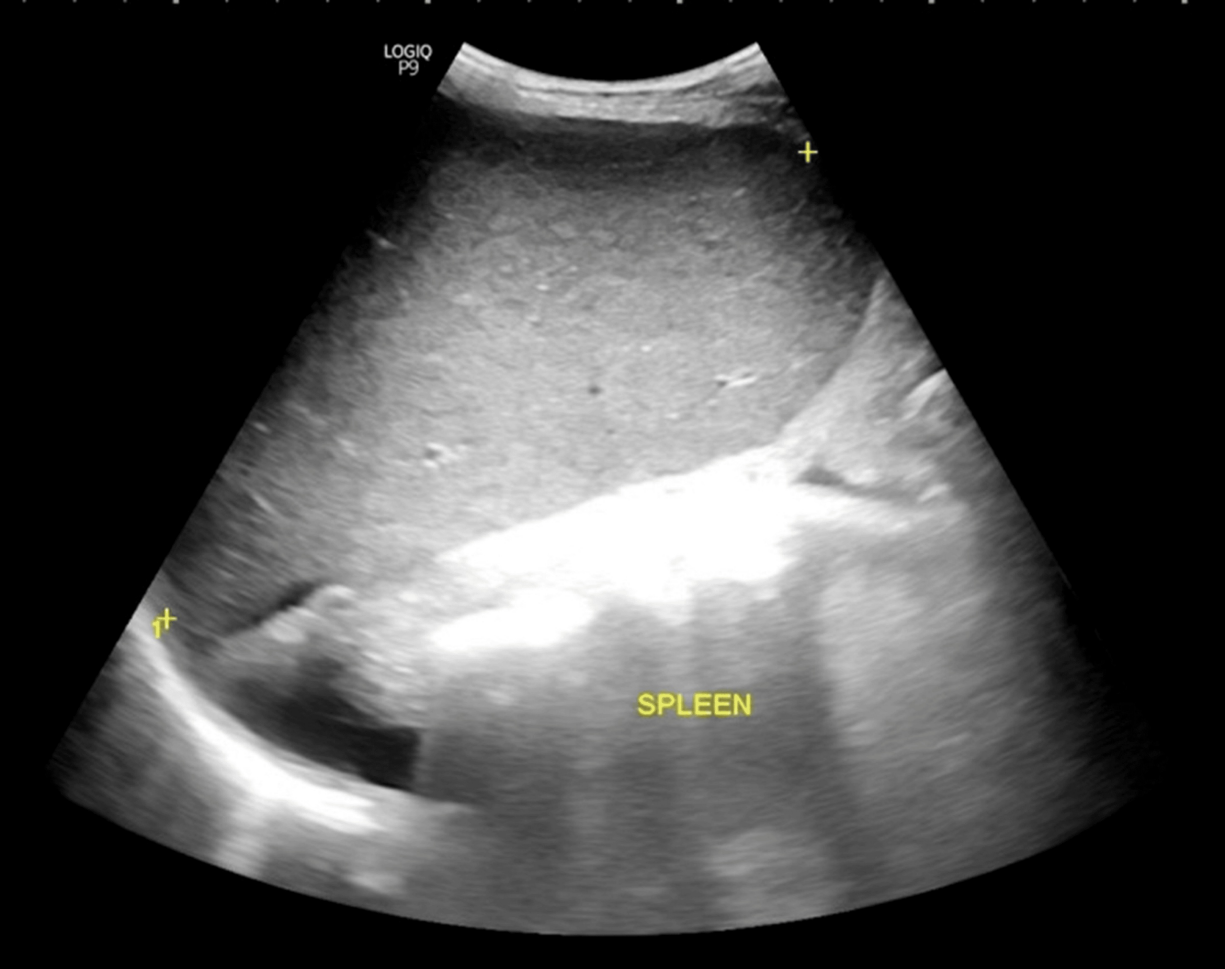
Ultrasound abdomen image of case 4. The image shows mild hepatomegaly and splenomegaly.
